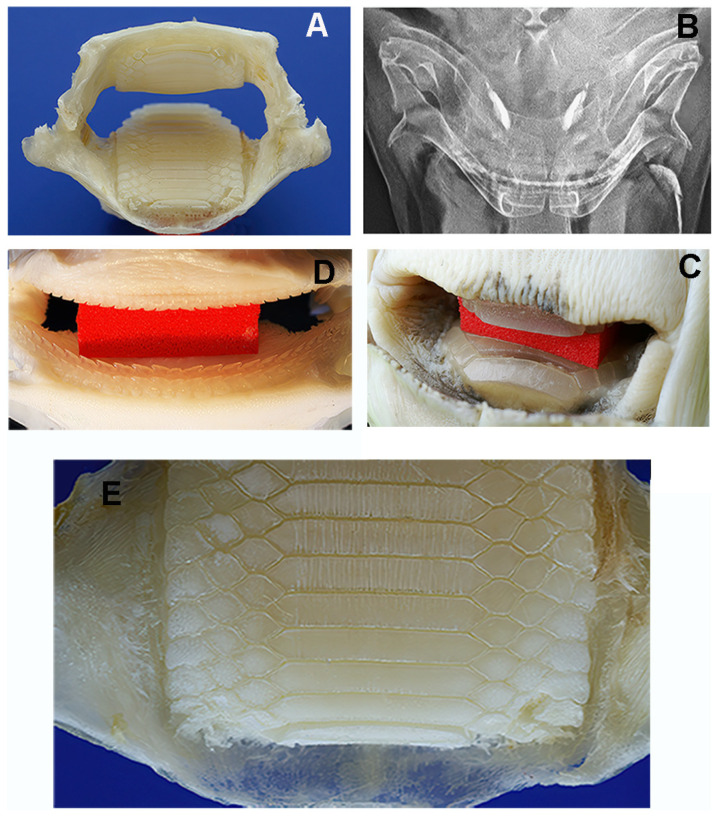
Myliobatis aquila*: morphology of the double masticatory system. (A,B) Dissection of maxilla and mandible before removal of the symphyseal plates (A) and X-rays of the head in dorso-ventral projection (B). The upper and lower jaw (thinner than that of *R. asterias*) are external to the symphyseal plates. The movement of the system (adduction/abduction) is based on the ball-socket joints at the extremities. (C) Red spacer inserted between the upper and lower plates. (D) Full view of aligned teeth lines on the mandibular arches after red spacer removal. (E) Detail of the longitudinal scratches on the lower plate corresponding to the contact zone (anterior) with the curved upper plate.
